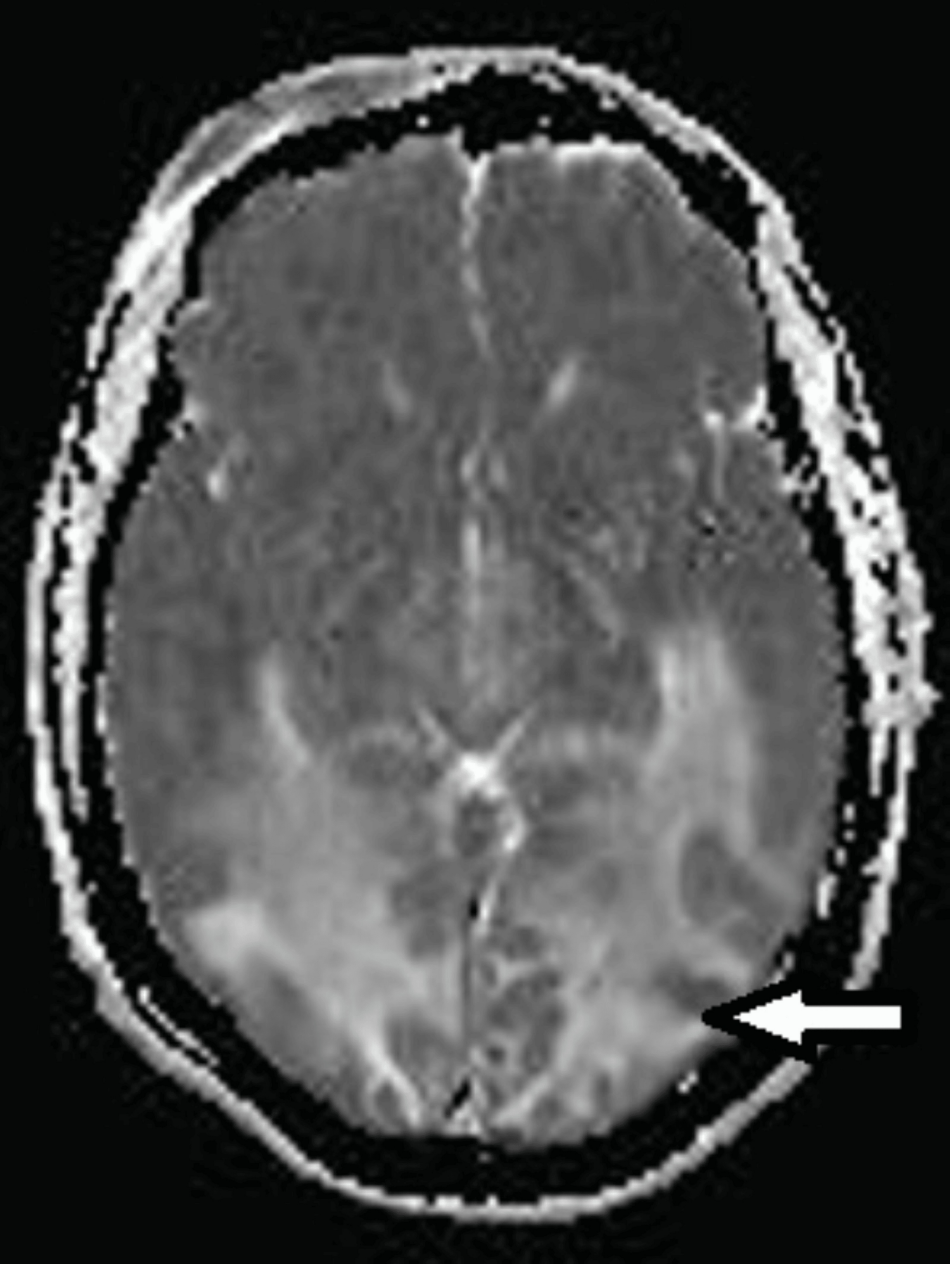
ADC Sequence on Magnetic Resonance Imaging in Case 1 The white arrow represents hypointensity, corresponding to the diffussion restriction on diffussion-weighted imaging. ADC: Apparent Diffussion Coefficient
